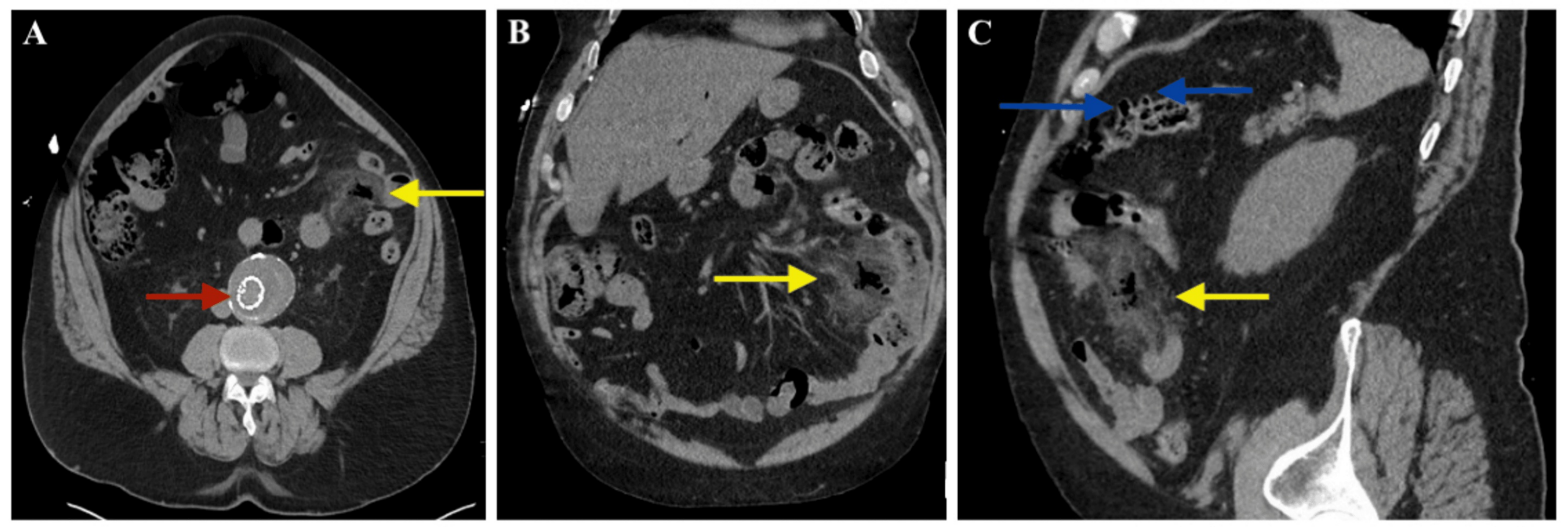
Abdominal computed tomography imaging Mesenteric inflammation and localized intramesenteric free air are indicated by yellow arrows (A-C). The stable abdominal aortic aneurysm repair is marked with a red arrow. Two small intestinal diverticula are visible (C, blue arrows).
